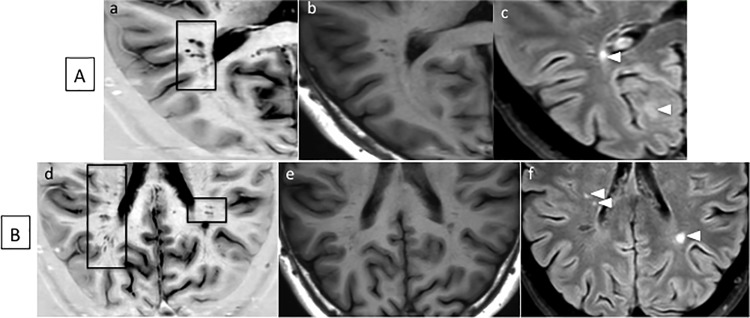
PSIR (a,d), T1 (b,e) and FLAIR (c, f) images of eVRS of two CIS patients (A, B). A high number of eVRS can be detected by PSIR even in patient at clinical onset and having low WM lesion load. Arrow heads indicate WM lesions. On FLAIR images (c,f) eVRS are easily distinguished from inflammatory lesions (hyperintense) for their hypointense signal. In patient (A), a cluster of at least five round-shaped eVRS is clearly visible on PSIR image (a, black rectangular frame) and is, although less clearly, visible on T1 and FLAIR images. In patient (B), many eVRS of various shapes can be observed on PSIR (d) while only a few of them are visible on T1 (e) or FLAIR (f) images, thus further confirming the superiority of PSIR in detecting eVRS.
